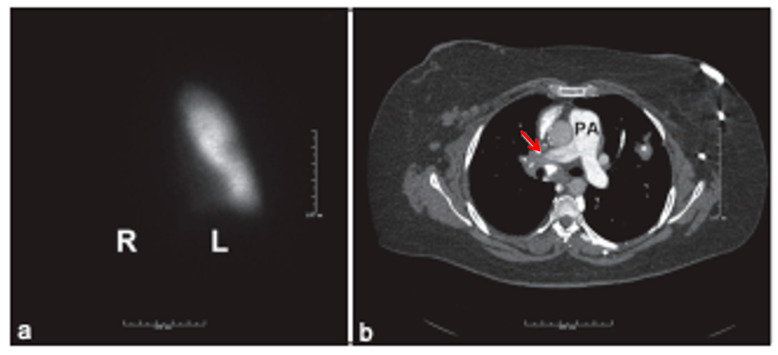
Ventilation perfusion scan and computed tomography angiography of a patient with pulmonary hypertension in the setting of fibrosing mediastinitis and absent flow to the right lung. (a) The lack of perfusion on the right lung. (b) An obstruction of the right main pulmonary artery (*red arrow*) due to fibrosing mediastinitis. R = right lung; L = left lung; PA = pulmonary artery. Reprinted/adapted with permission from Ref. [84].
